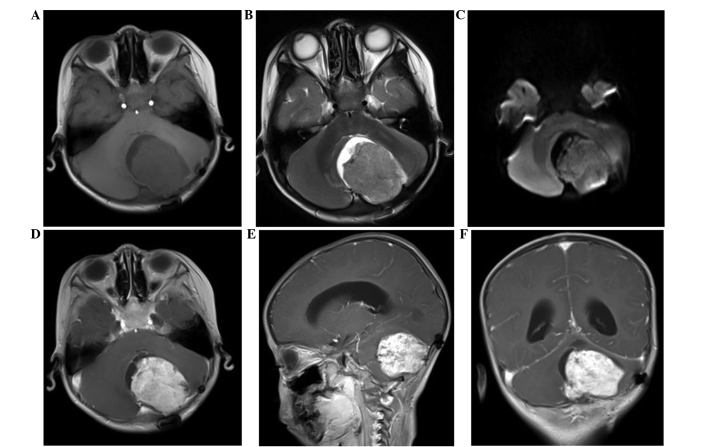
Two months after the surgery, magnetic resonance imaging of the brain on (A) T1WI, (B) T2WI, (C) diffusion-WI and (D-F) T1WI with gadolinium suggesting that the tumor had increased in size following transient expansion. W1, weighted imaging.
